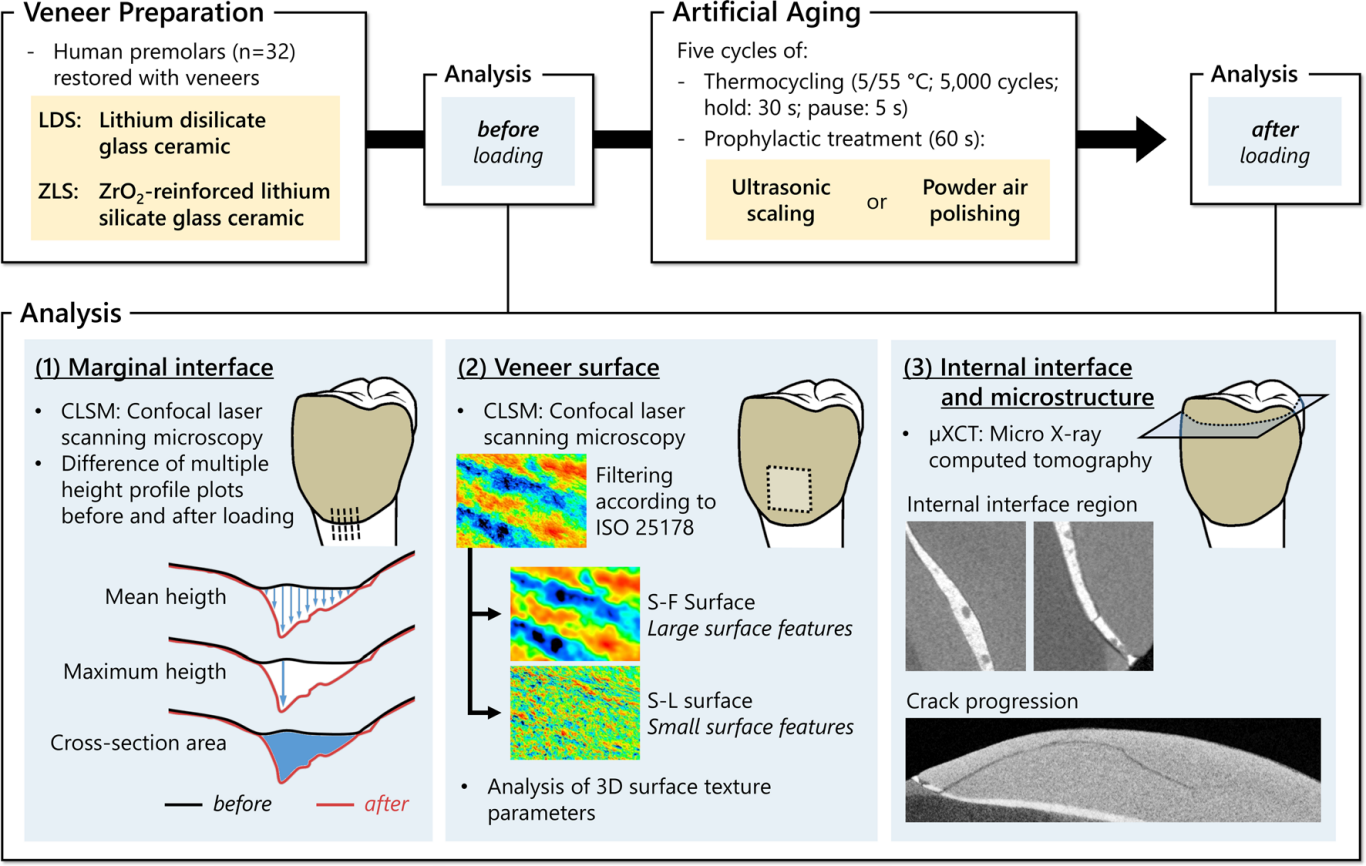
Study design and methodology. The factors of the two-way ANOVA (yellow) were the restorative material (LDS, ZLS) and the prophylaxis treatment (ultrasonic scaling and powder air polishing)
